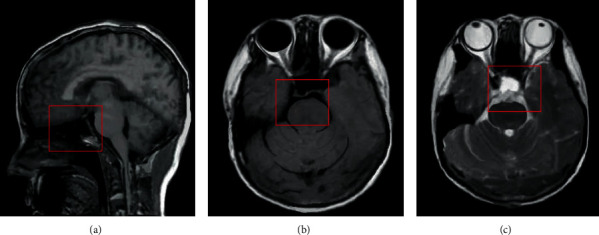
MRI images of a patient.
